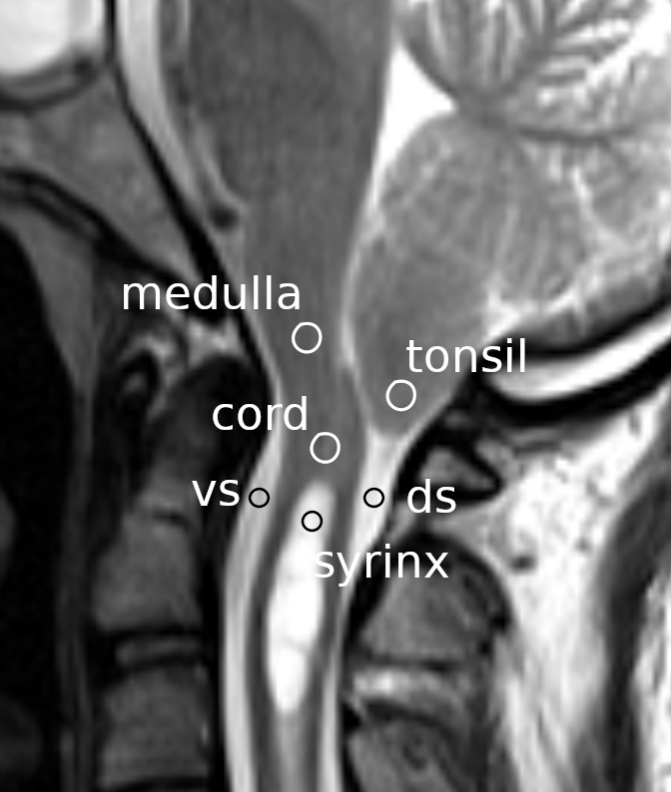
Regions of interest measured. Circles indicate the regions of interest on a mid-sagittal section of a magnetic resonance image at the craniovertebral junction. vs: ventral subarachnoid space; ds: dorsal subarachnoid space.
